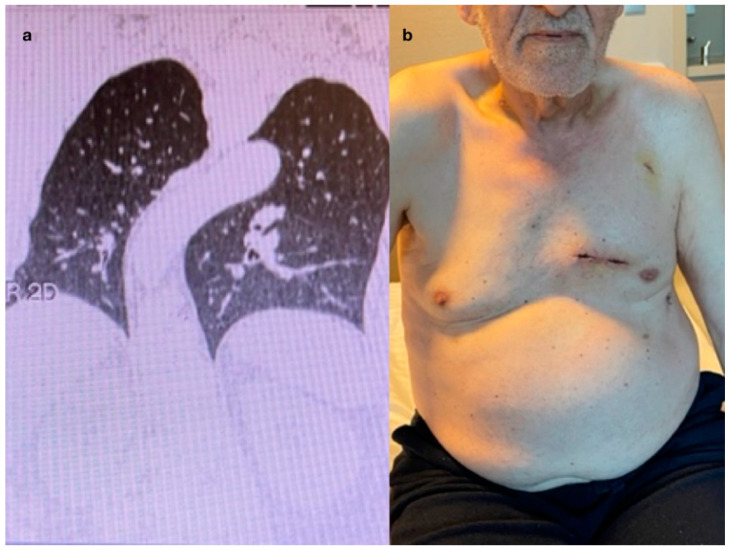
(a) A coronal section from the preoperative CT of the patient with severe kyphoscoliosis, and (b) the wound in the first week postoperatively can be seen.
